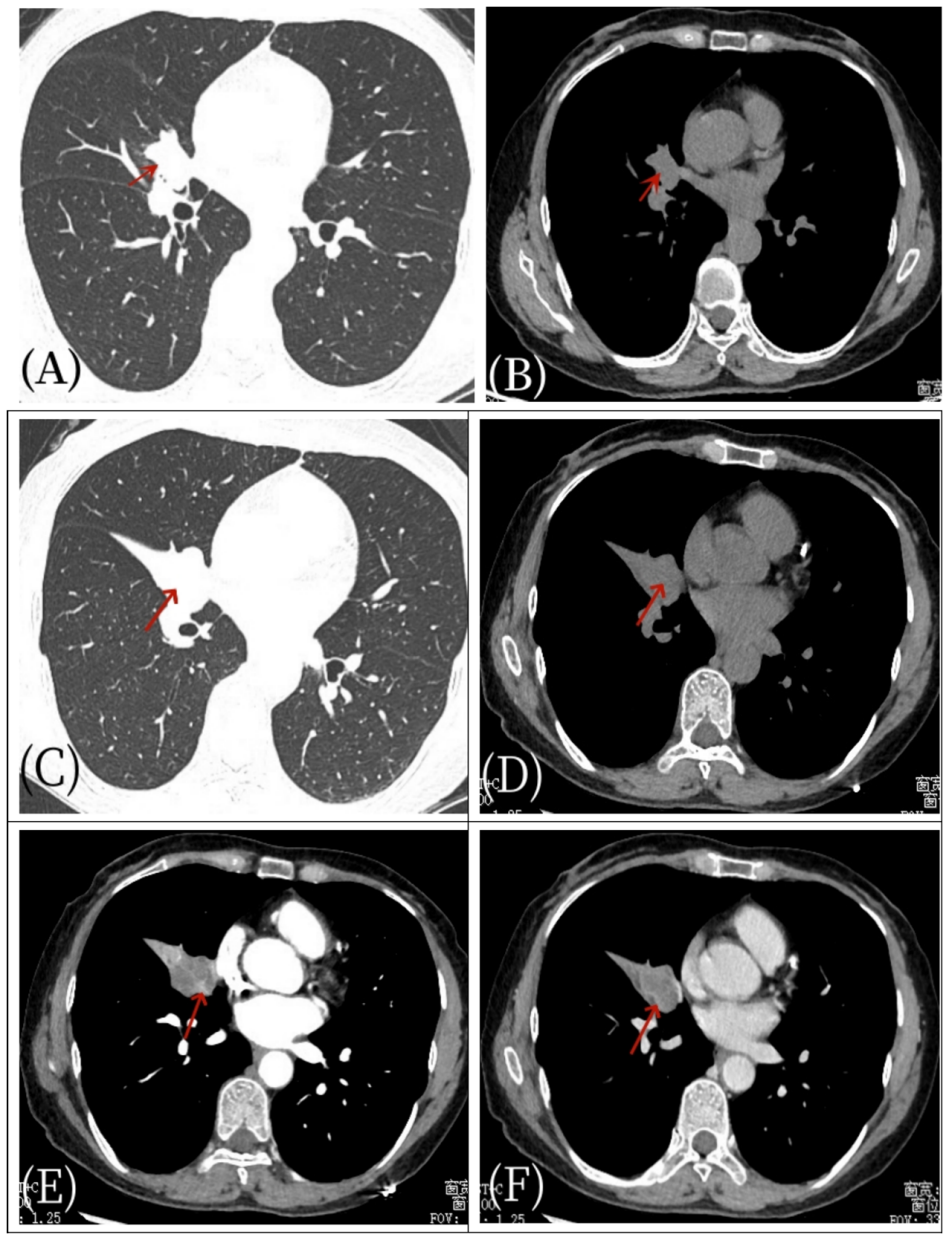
Female, 57 years old, pulmonary angiomatoid fibrous histiocytoma. On April 27, 2021, a non-contrast CT (A,B) scan showed a space within the bronchus of the right middle lobe (arrow) without evidence of obstructive atelectasis or pneumonia. After 1 week, the patient’s condition worsened, manifesting as pulmonary atelectasis. Subsequent axial non-contrast CT scan (C,D) and axial CT enhanced scan (E,F) revealed right middle lobe bronchial obstruction, atelectasis with a soft tissue density shadow (arrow), and significant enhancement of the mass (arrow) as well as mediastinal enlarged lymph node shadows.
